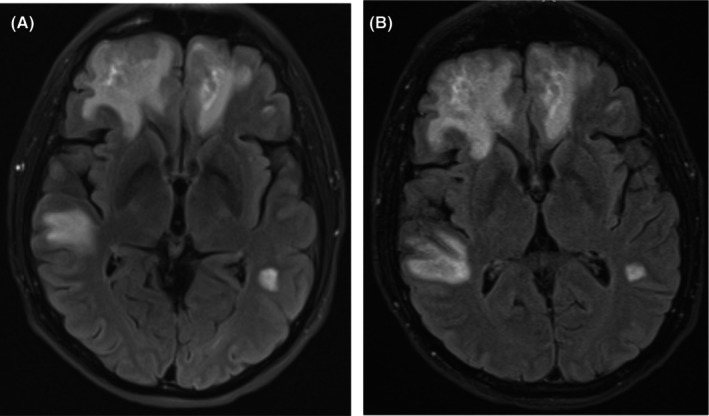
Slices from T2‐weighted fluid‐attenuated inversion recovery (FLAIR) sequences from MRI brain from hospital Day 1 (A) and hospital Day 13 (B) demonstrating abnormal white matter signals bilaterally.
